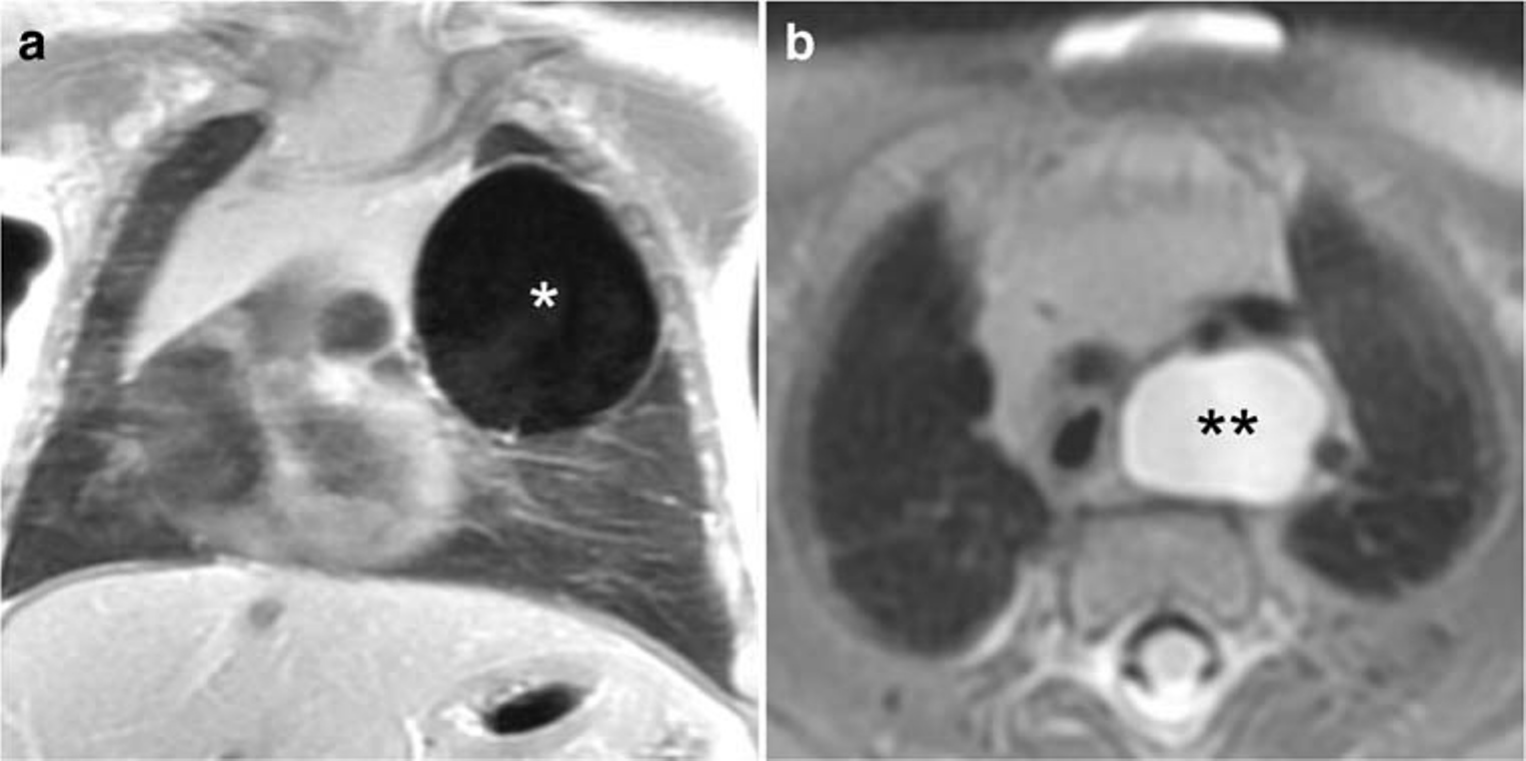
Bronchogenic cysts in an 8-week-old boy. a Coronal proton density MR image shows a single air-filled cyst (*) in the left upper lobe following thoraco-amniotic drainage in utero. b Axial T2-weighted image shows a second mediastinal fluid-filled cyst () with connection to the tracheal wall
